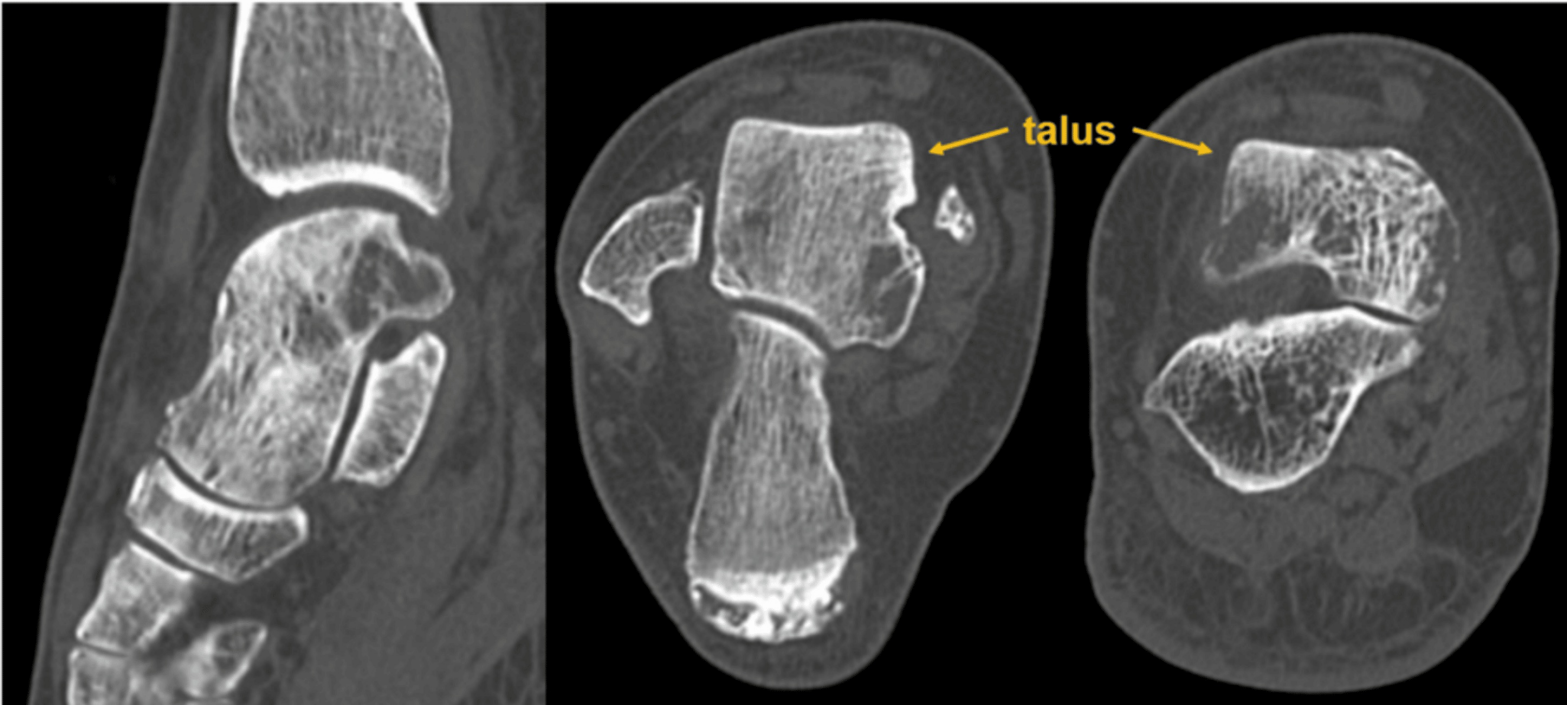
Computed Tomography (CT) scans showed coarse cancellous bone in the entire right talus and some cystic changes.
